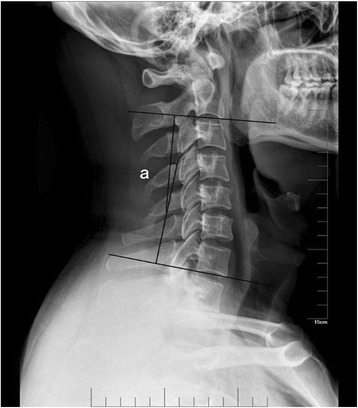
Lateral radiograph showing cervical lordosis (Cobb angle of C2–C7) calculated by measuring the angle formed by the lines along the inferior endplate of C2 to the inferior endplate of C7 in the neutral position
